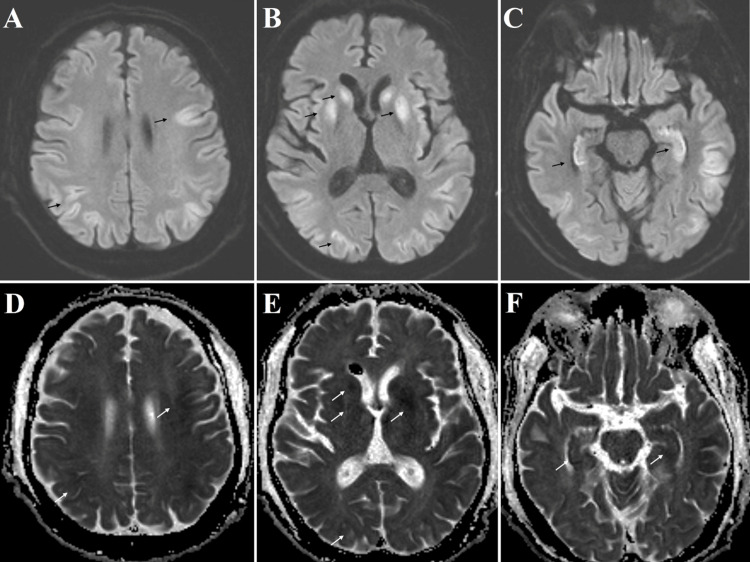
Magnetic resonance imaging with diffusion-weighted imaging (A-C) sequences and apparent diffusion coefficient maps (D-F) demonstrating numerous areas of diffusion restriction (white and black arrows) in the cortex, hippocampus, and basal ganglia consistent with anoxic brain injury
